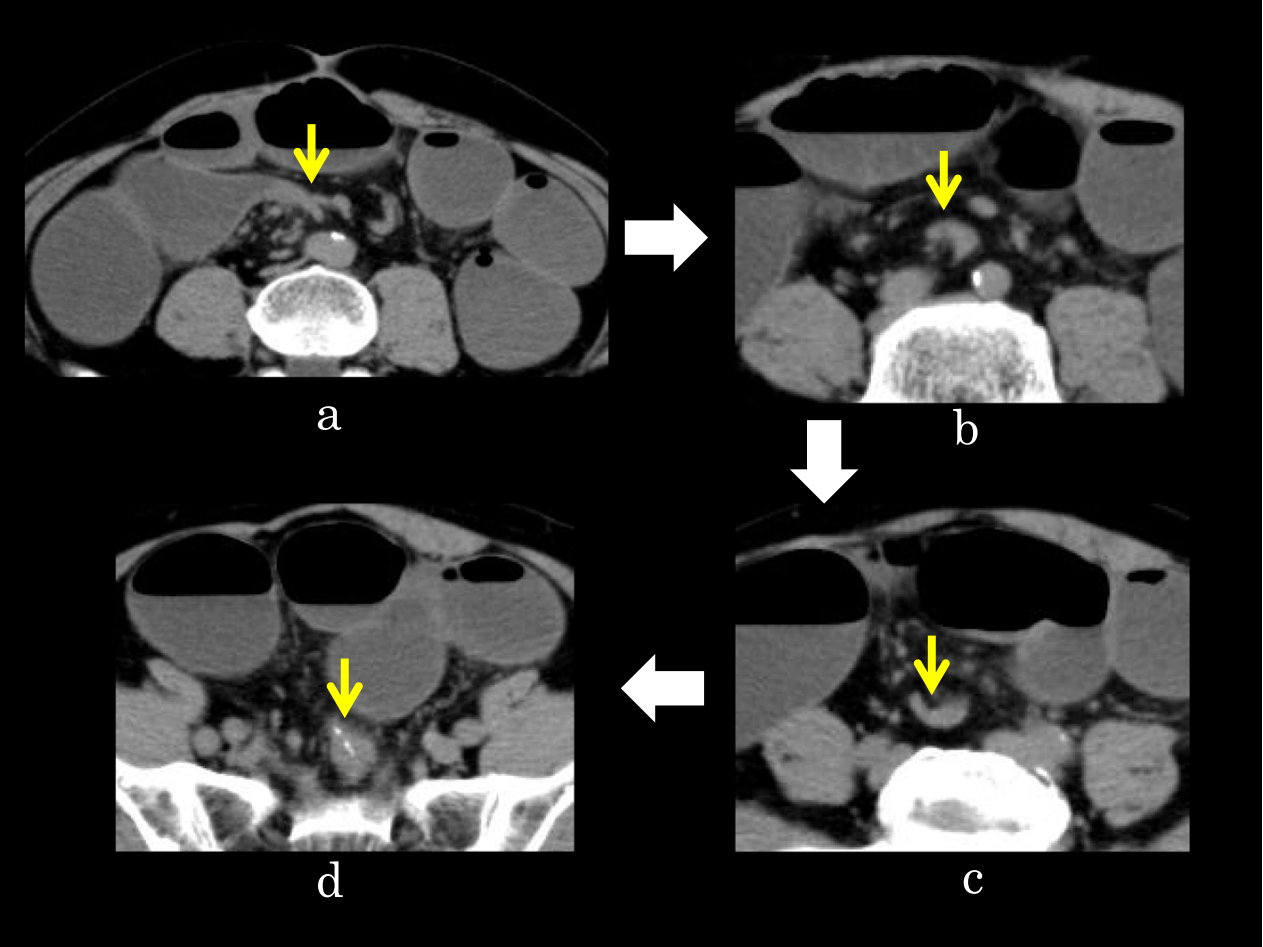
CT of the SBO. The ileal stricture had a beak-like appearance (a, yellow arrow). The ileum became narrower on the anal side and was twisted (b, c, yellow arrows), then it connected to the ileal pouch (d, yellow arrow)
